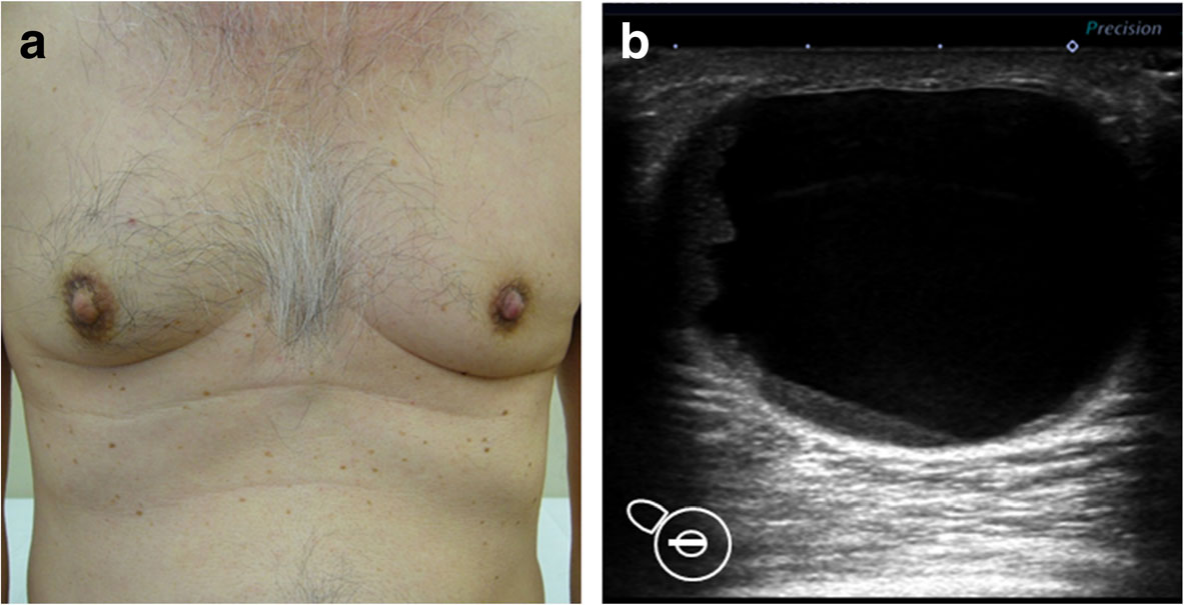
Physical and ultrasonography findings: An elastic and soft neoplastic 3-cm lesion was palpated in the right papilla (a). On mammary ultrasonography, a cystic lesion of 3.9 × 2.6 cm was confirmed immediately below the right papilla (mixed pattern). As a 1-cm solid tumor with a gradual rise from the cyst wall was confirmed within the cyst (b)
